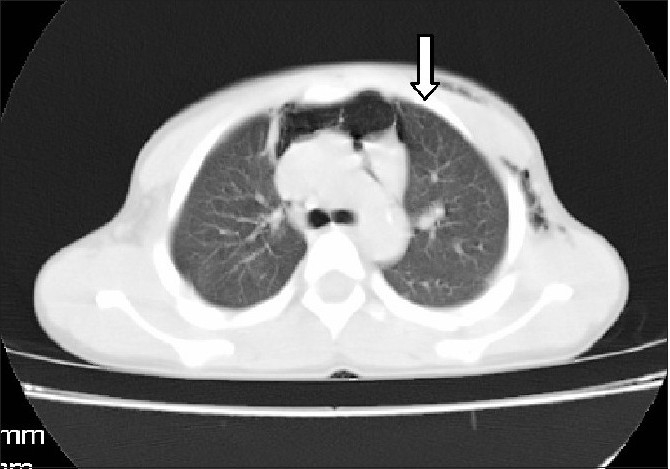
CT picture showing pneumomediastinum (white arrow)
